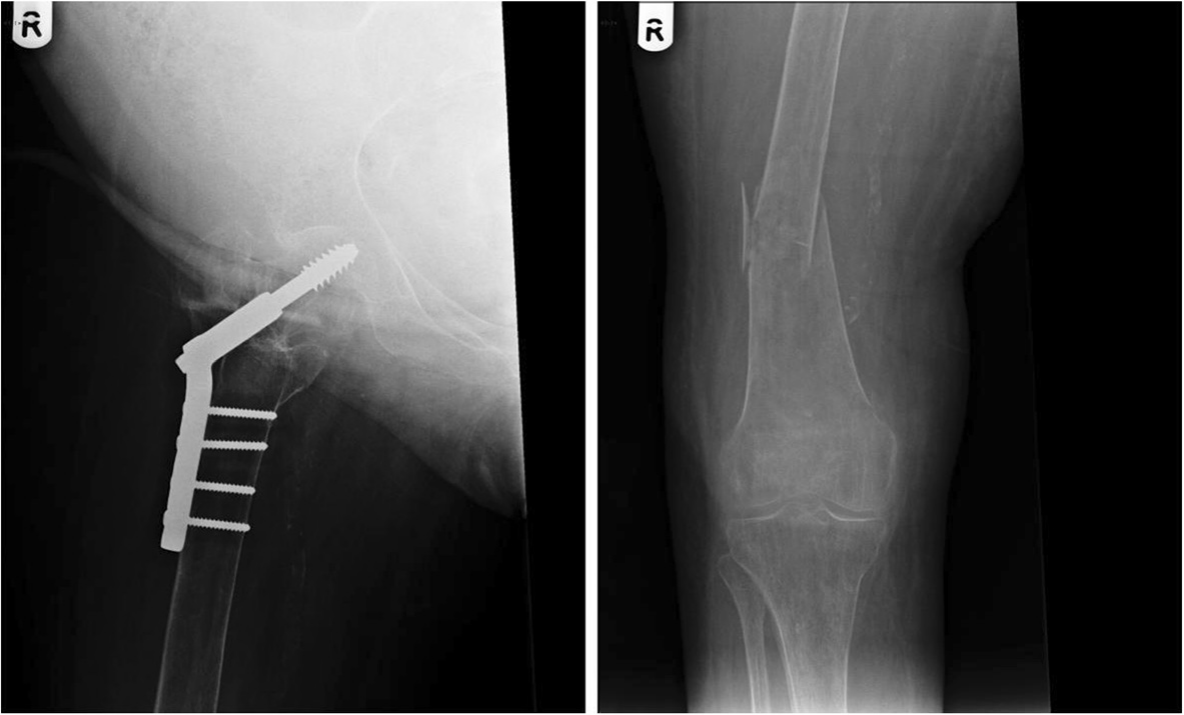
Left panel: X-ray of right thigh of patient #3 revealed previous dynamic hip screw internal fixation right hip. Right panel: X-ray of right thigh and knee of patient #3 revealed fracture distal third shaft of femur with mild varus angulation.
